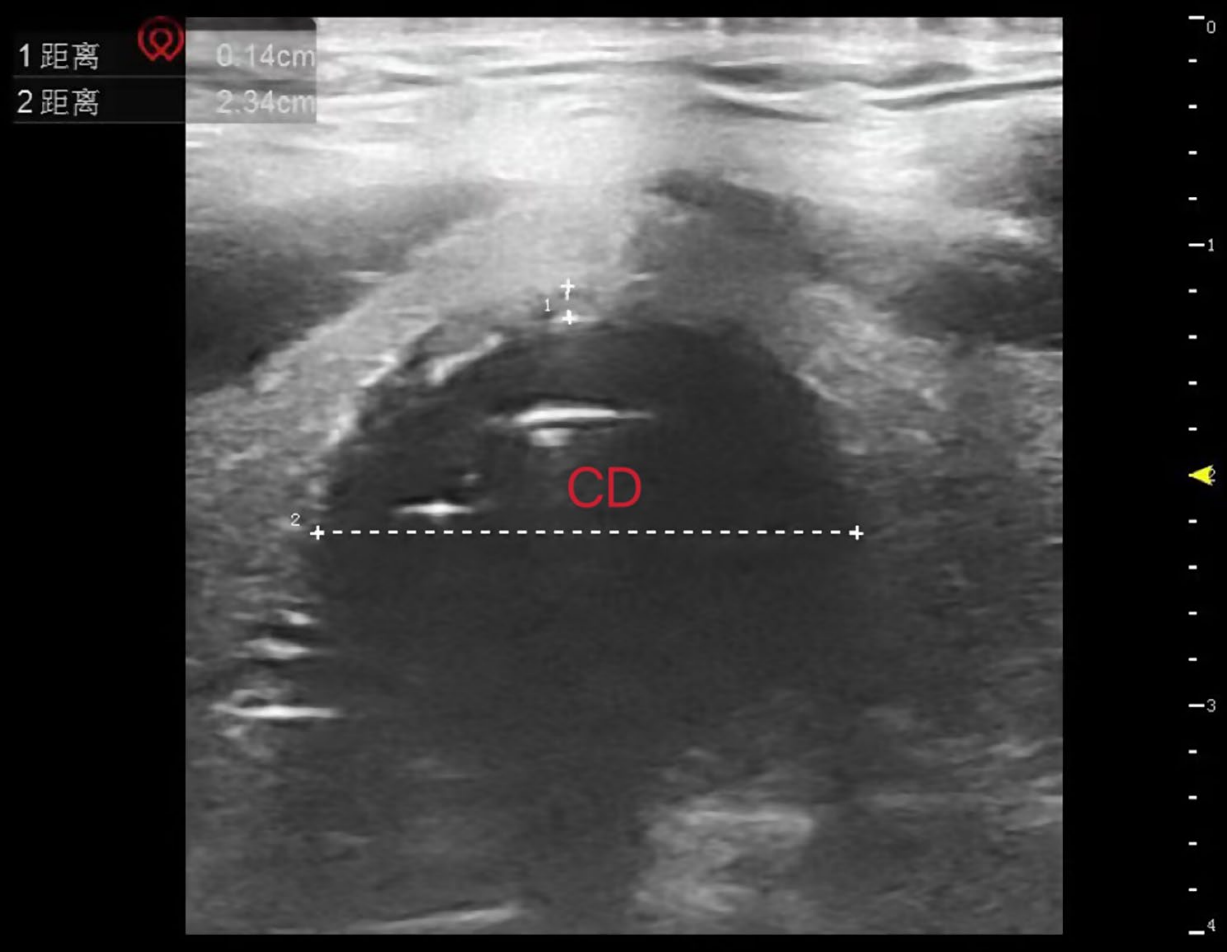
The CD at the level of the suprasternal notch measured by ultrasound. To find the maximal diameter of the tracheal tube cuff, we first placed the probe on the level of the suprasternal notch to confirm the placement of the tracheal tube cuff, then slowly moved the probe upright toward the head to find the maximal diameter of the tracheal tube cuff. The shadow: cuff; CD: tracheal tube cuff transverse diameter
